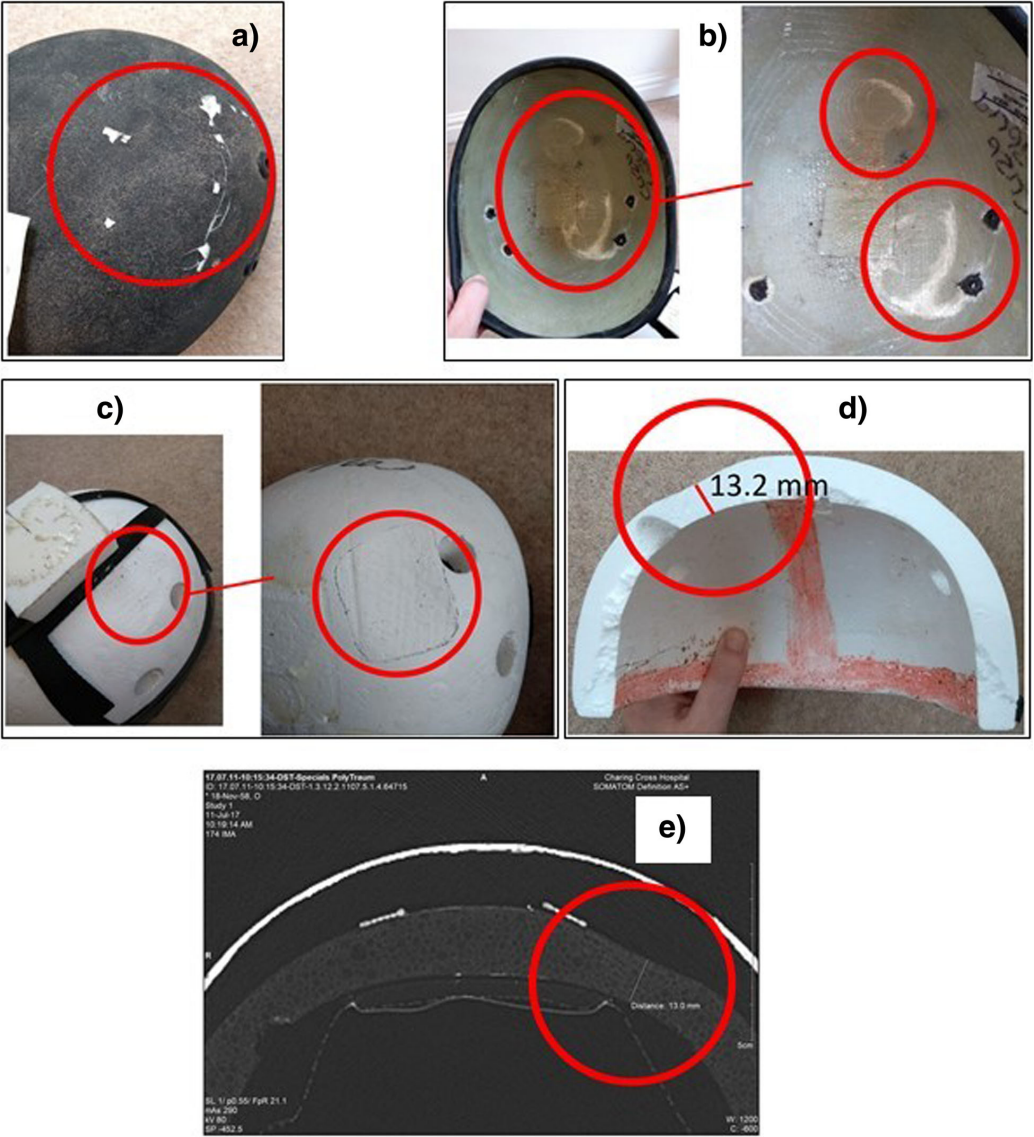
Example, identifying helmet damage. a Visible cracks can be seen on the outer surface of the fibreglass shell. One area of damage can be seen. b Visible delamination of the fibreglass shell can be seen in two distinct locations. Top and bottom of photo. The damaged area at the bottom of the photo corresponds to the external damage. c Visible crush of the EPS liner. d A section was cut through the centre of the damaged area and the liner thickness was measured at its thinnest point. It measured 13.2 mm. e Reviewing the CT images showed clear crushing of the EPS liner. At its thinnest point, the crushed region measured 13 mm in this case
